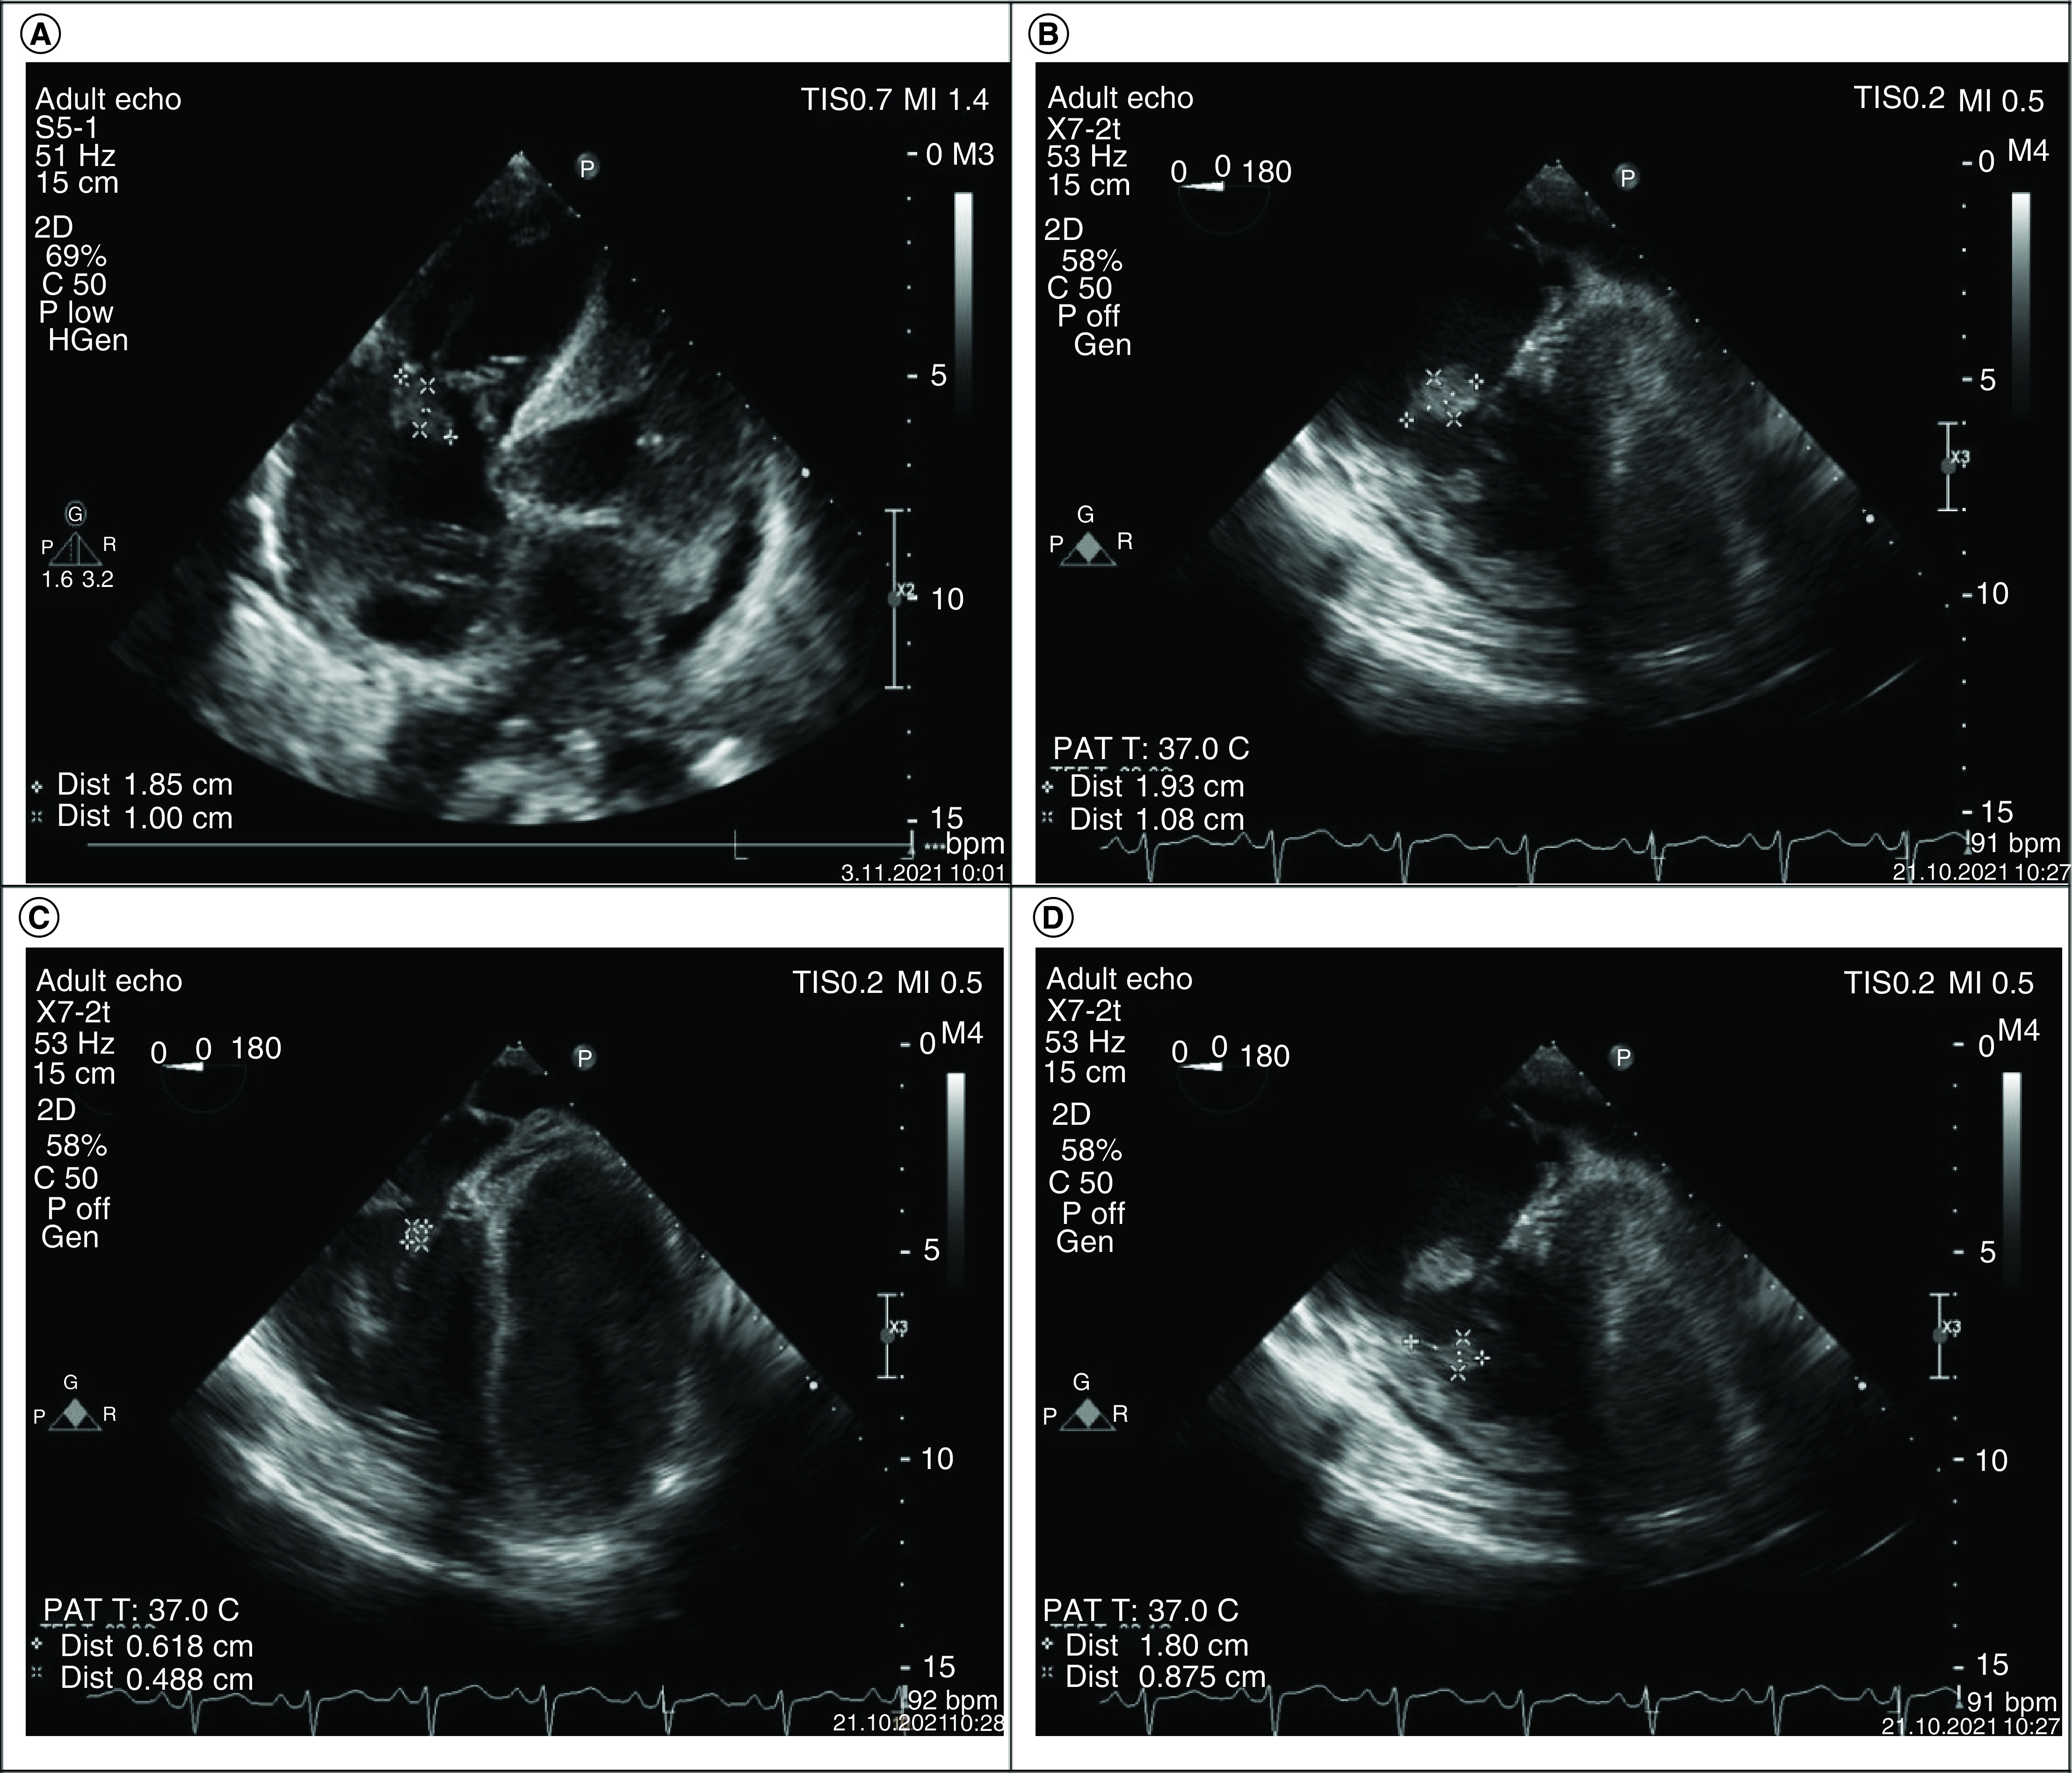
Transthoracic and transesophageal echocardiography images of the patient.
